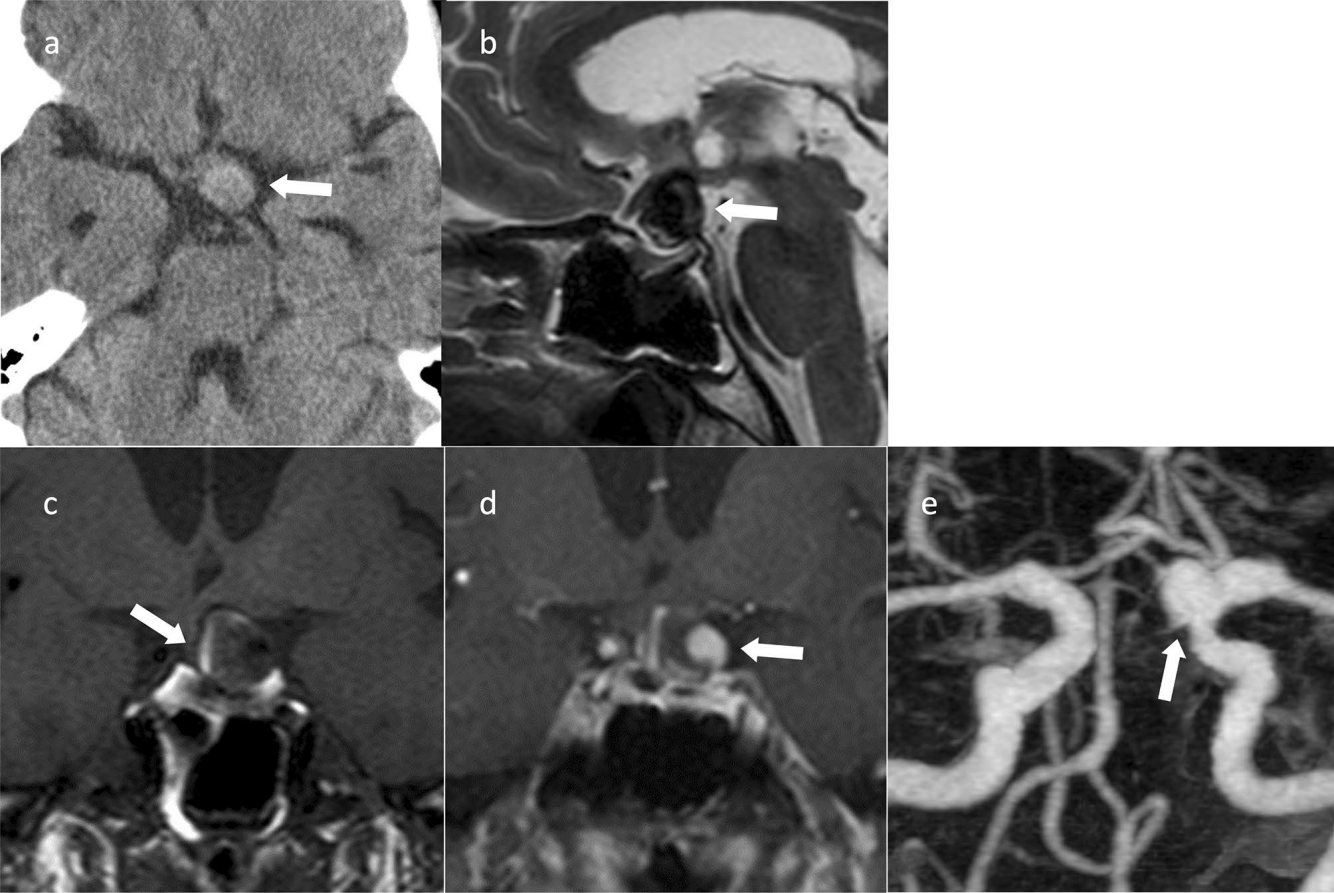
Internal carotid-posterior communicating artery aneurysm with partial thrombosis. An approximately 70-year-old female with headache. a Computed tomography shows a high-density mass in the suprasellar region (arrow). b Sagittal T2WI shows a hypointensity mass above the sella turcica (arrow). c Coronal T1WI shows hypointensity in the mass but with a hyperintense area at the limbus, indicating a thrombus (arrow). d Coronal contrast-enhanced T1WI shows a nodular area with contrast enhancement in the mass (arrow). e Magnetic resonance angiography shows an aneurysm in the posterior communicating artery of the right internal carotid artery (arrow)
